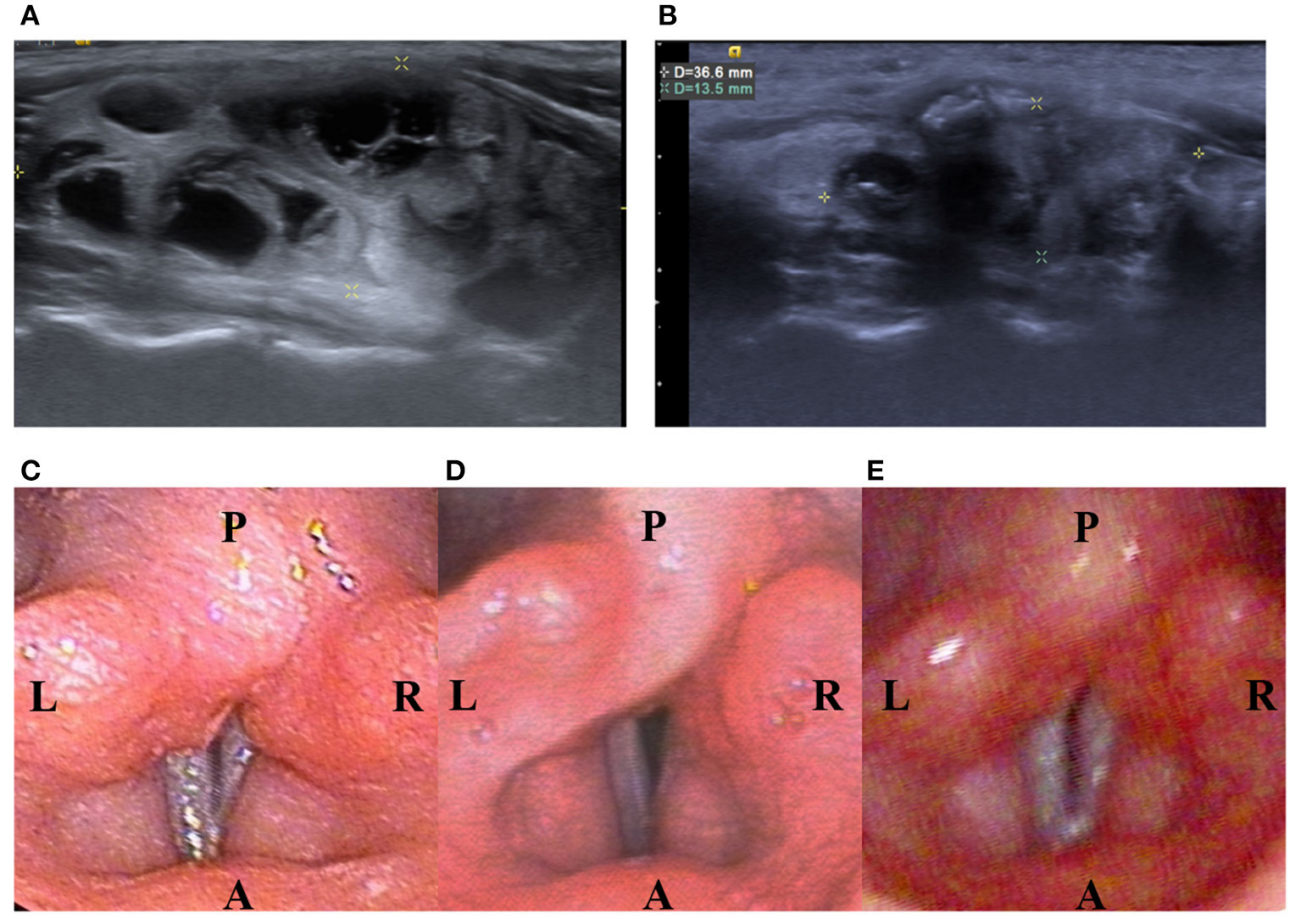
A 13-year-old female patient with a palpable nonfunctioning thyroid mass. (A) Before RF ablation, longitudinal sonographic views predominantly solid nodules in the right thyroid lobe. Initial nodule volume was 22.32 mL. (B) At 6 months after the ablation, a longitudinal sonographic view showed that the right thyroid nodule had shrunk. Nodule volume at 6-month follow-up was 4.65 mL, a volume reduction rate of 83%. (C) Before RF ablation, laryngoscope showed normal vocal cord. (D) On the same day after RF ablation, laryngoscope showed relative weakness of the right vocal cord but still with positive vibration. (E) On the 53rd day after RF ablation, laryngoscope showed normal vibration of bilateral vocal cord.
